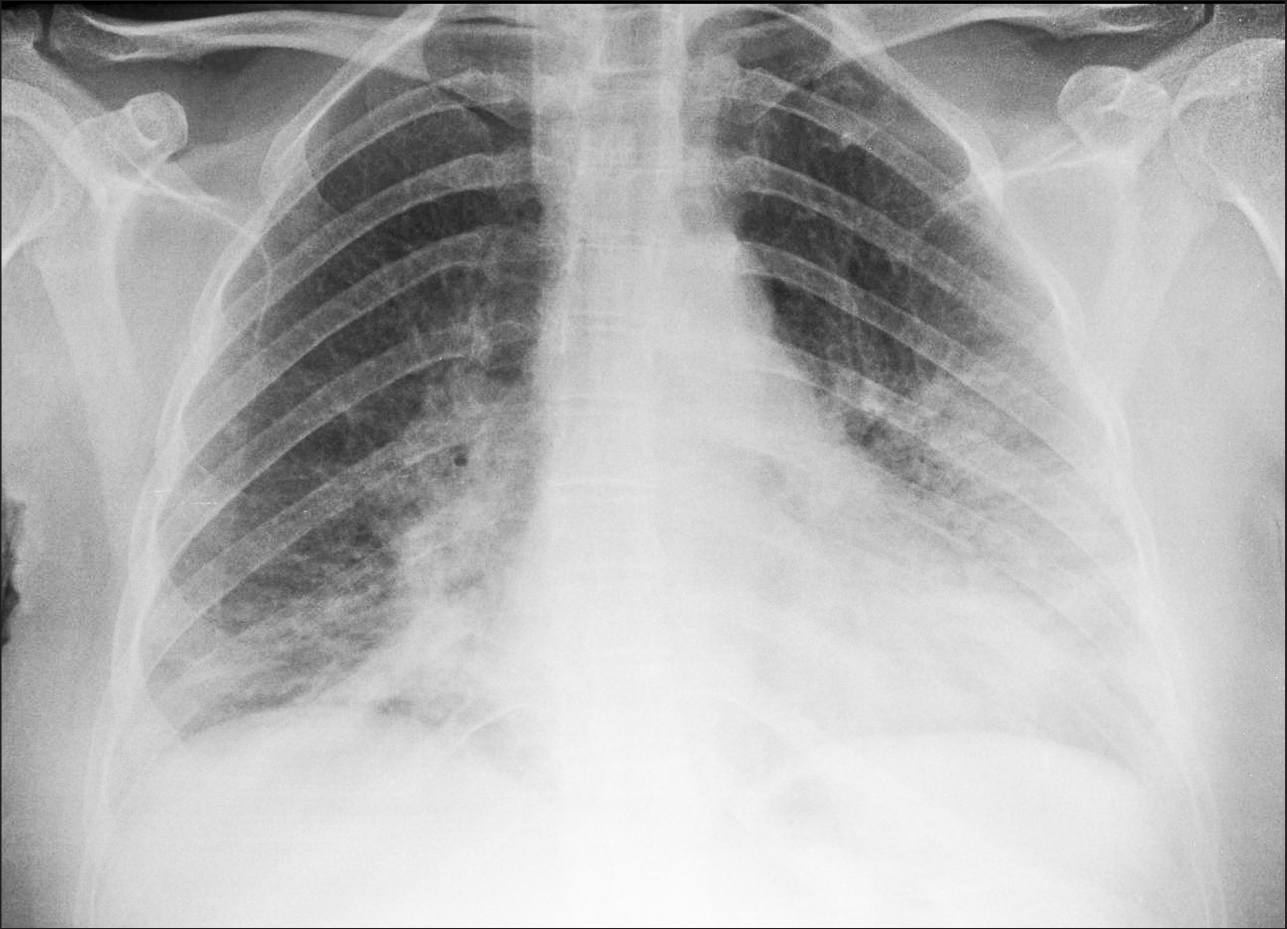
Anteroposterior bedside chest X-ray showing GGO and consolidation in a patient presenting to the emergency department with ARDS. Chest HRCT showed no pleural effusion.
